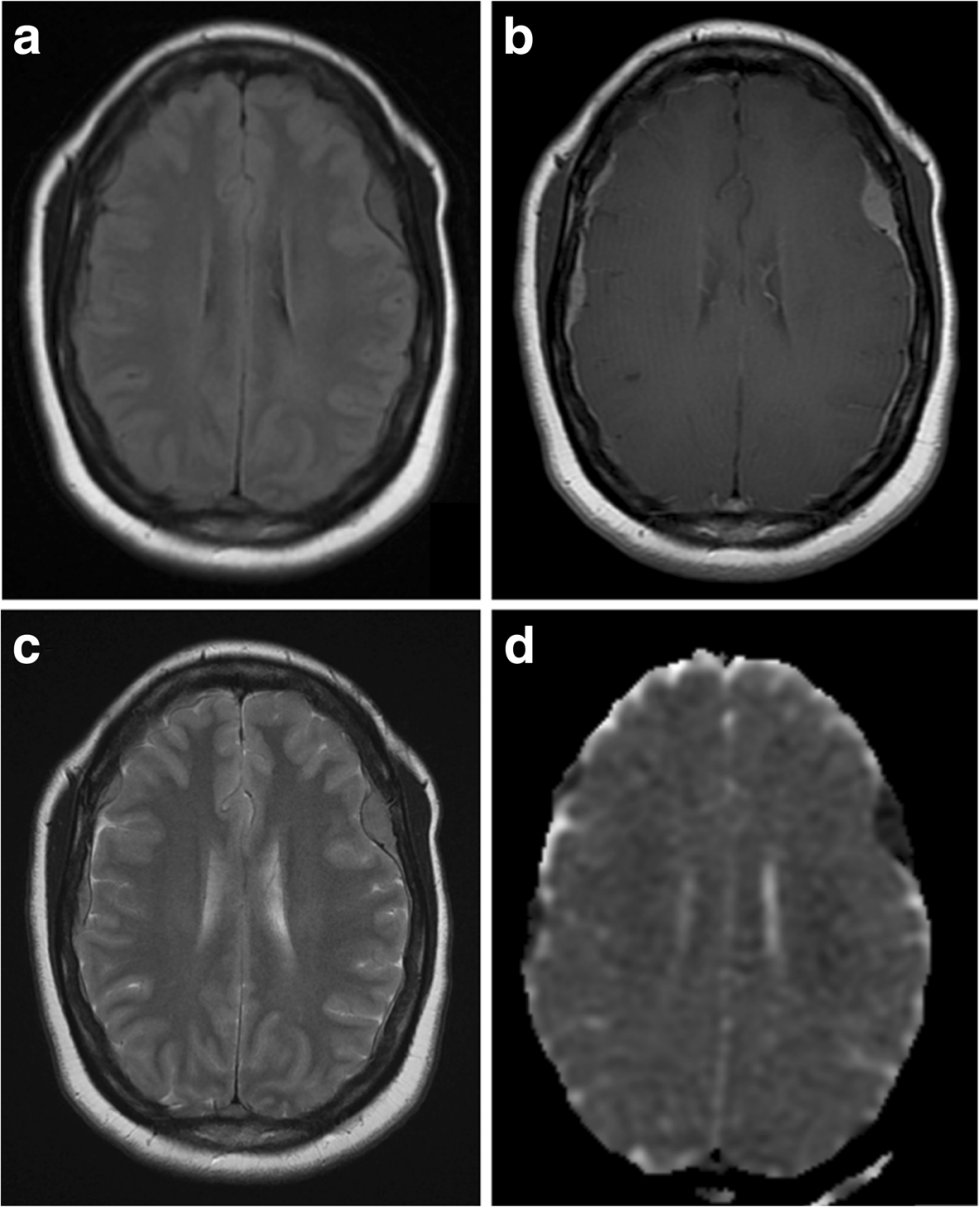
Multifocal dural lymphoma. a, b Pre- and post-contrast axial T1-weighted MR images showing diffuse dural thickening and enhancement with superimposed dural masses. c Axial T2-weighted MR image of the same lesions. d Apparent diffusion coefficient (ADC) map showing intense restricted diffusion within the multiple dural mass lesions in keeping with high cellularity
